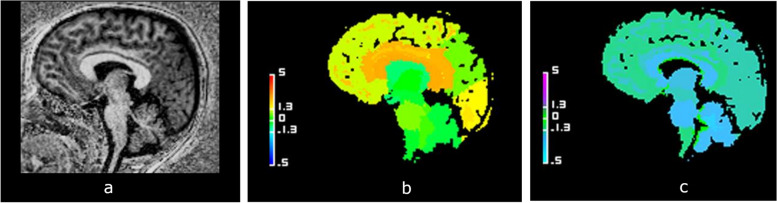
Example of hypertrophic corpus callosum (CC). Sagittal views of the brain midline in a 44-month-old girl with neurodevelopmental delay. a High-contrast anatomical T1-weighted uniform image showing a hyperplasia of both the corpus callosum and the sus tentorial stage. b Abnormal volumetric deviation map, with a CC appearing in orange, *Z*-score = 3.18. c Decreased T1 relaxometric deviation map, with a CC appearing in blue, *Z*-score = -2.40
